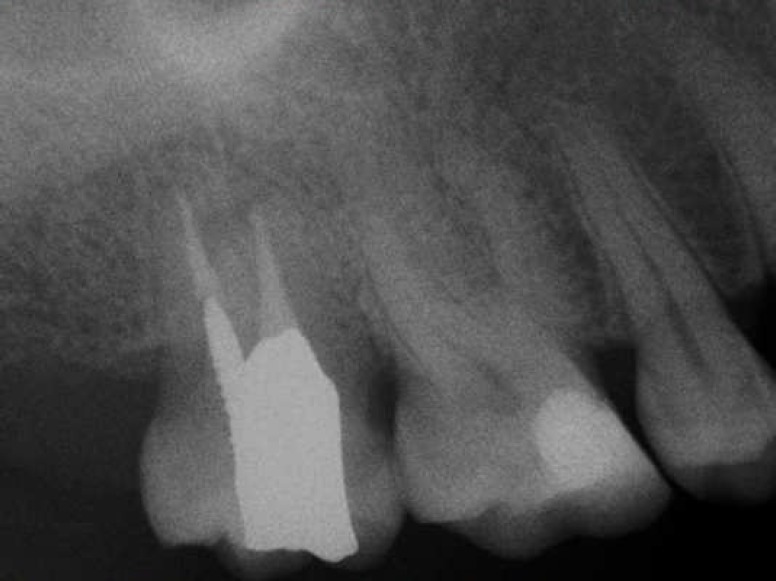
Recall radiograph revealed complete healing of periapical lesion
